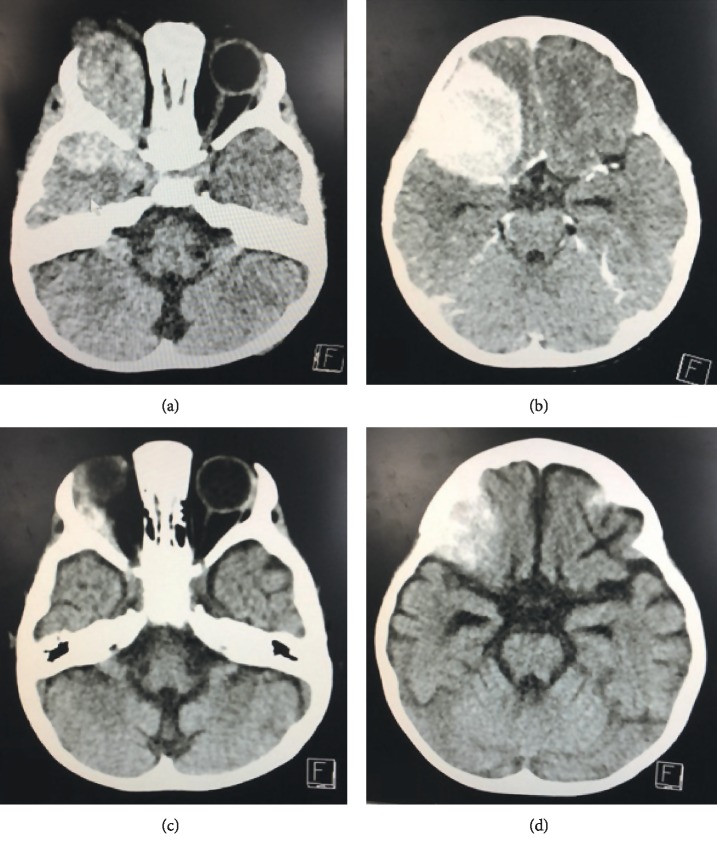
(a, b) Computed tomography scan revealed a homogeneous enhancing mass involving the right orbit with intracranial extension. (c, d) After 4 weeks of induction chemotherapy, decrease in the size of homogeneous enhancing mass involving the right orbit with intracranial extension.
